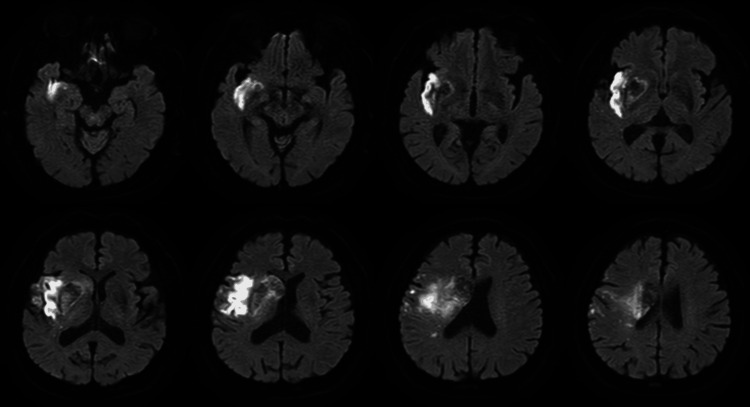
MRI at the time of onset showing hemorrhagic cerebral infarction in the region of the right middle cerebral artery.
